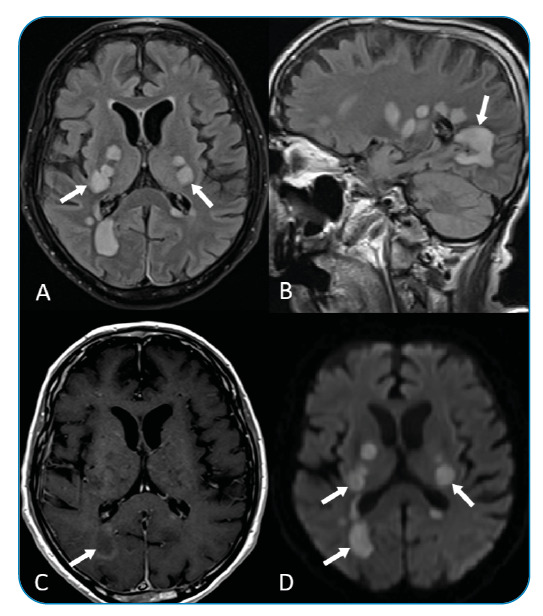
Fluid-attenuated inversion recovery magnetic resonance imaging (MRI) findings in the (A) axial and (B) sagittal views showing multiple hyperintense white matter lesions and basal nuclei bilaterally (arrows). (C) Contrast-enhanced T1-weighted MRI findings showing an incomplete arc of contrast enhancement (arrows). (D) MRI diffusion-weighted imaging showing hyperintense lesions (arrows).
